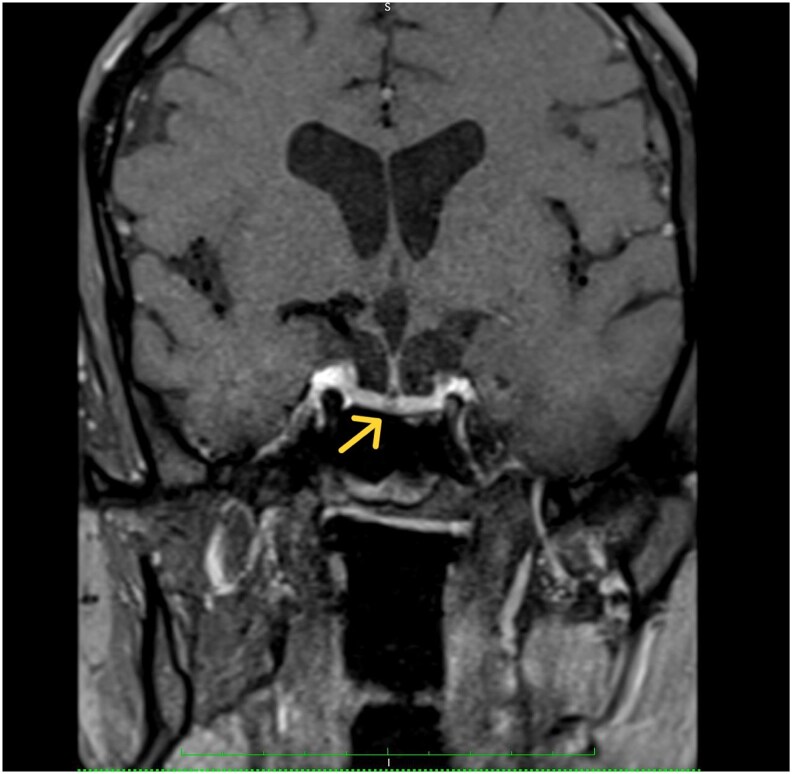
Magnetic resonance imaging of the pituitary showing a nonenhancing microadenoma.
